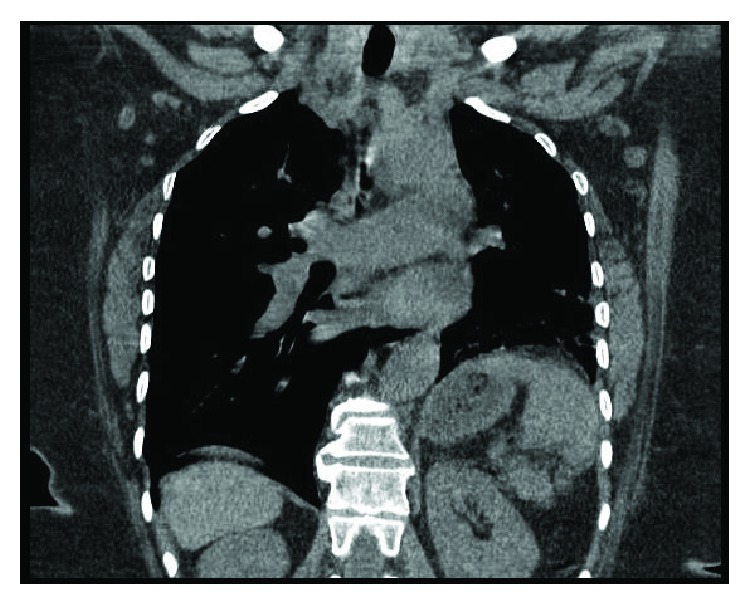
Coronal CT chest demonstrated marked enlargement of the right pulmonary artery with absence of the left pulmonary artery.
